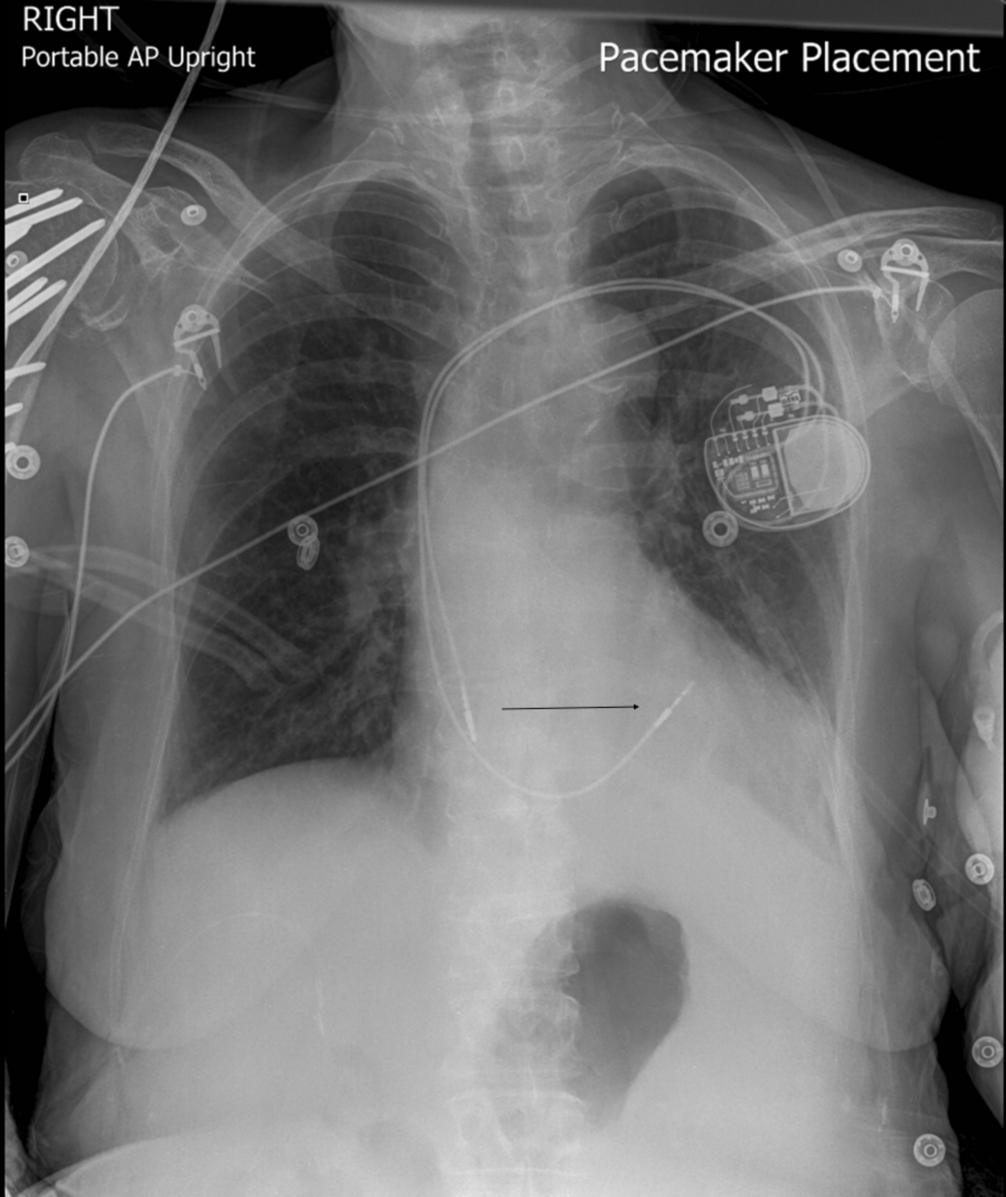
Chest X-ray of repositioned lead This image shows the repositioned pacemaker lead.
